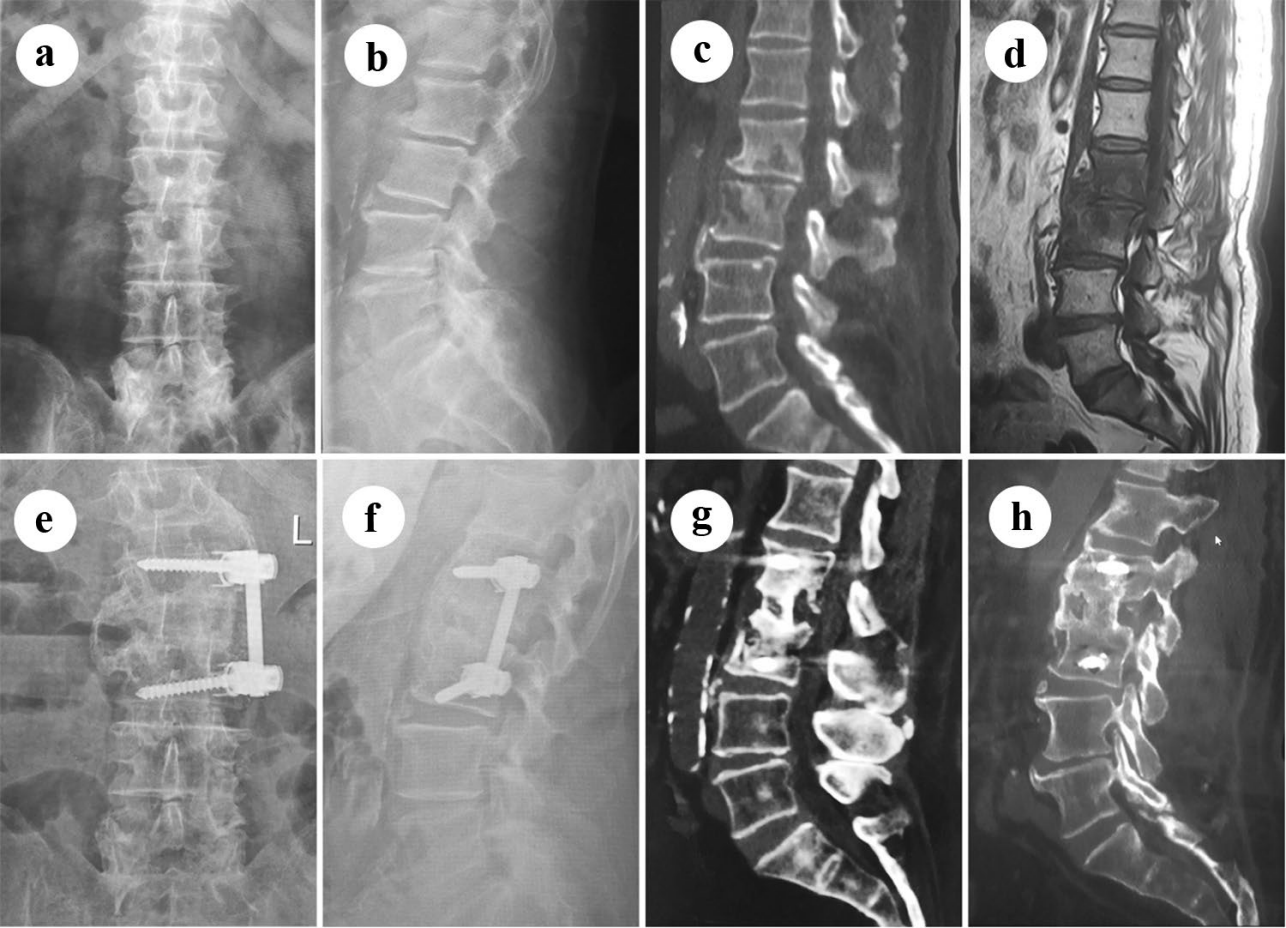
A 73-year-old male patient with L2–3 mono-segmental spinal TB, who complained of severe back pain for 7 months, received single anterior debridement, interbody fusion and vertebral fixation. a, b Preoperative X-ray of AP and lateral images show lumbar degeneration and loss of L2-3 intervertebral height. c, d Preoperative CT and MRI images show the destruction of vertebral bodies and disc. e, f Postoperative X-ray of AP and lateral images show good position of vertebral screw fixation. g, h Postoperative CT images show solid interbody fusion had been achieved at the postoperative 24th month
